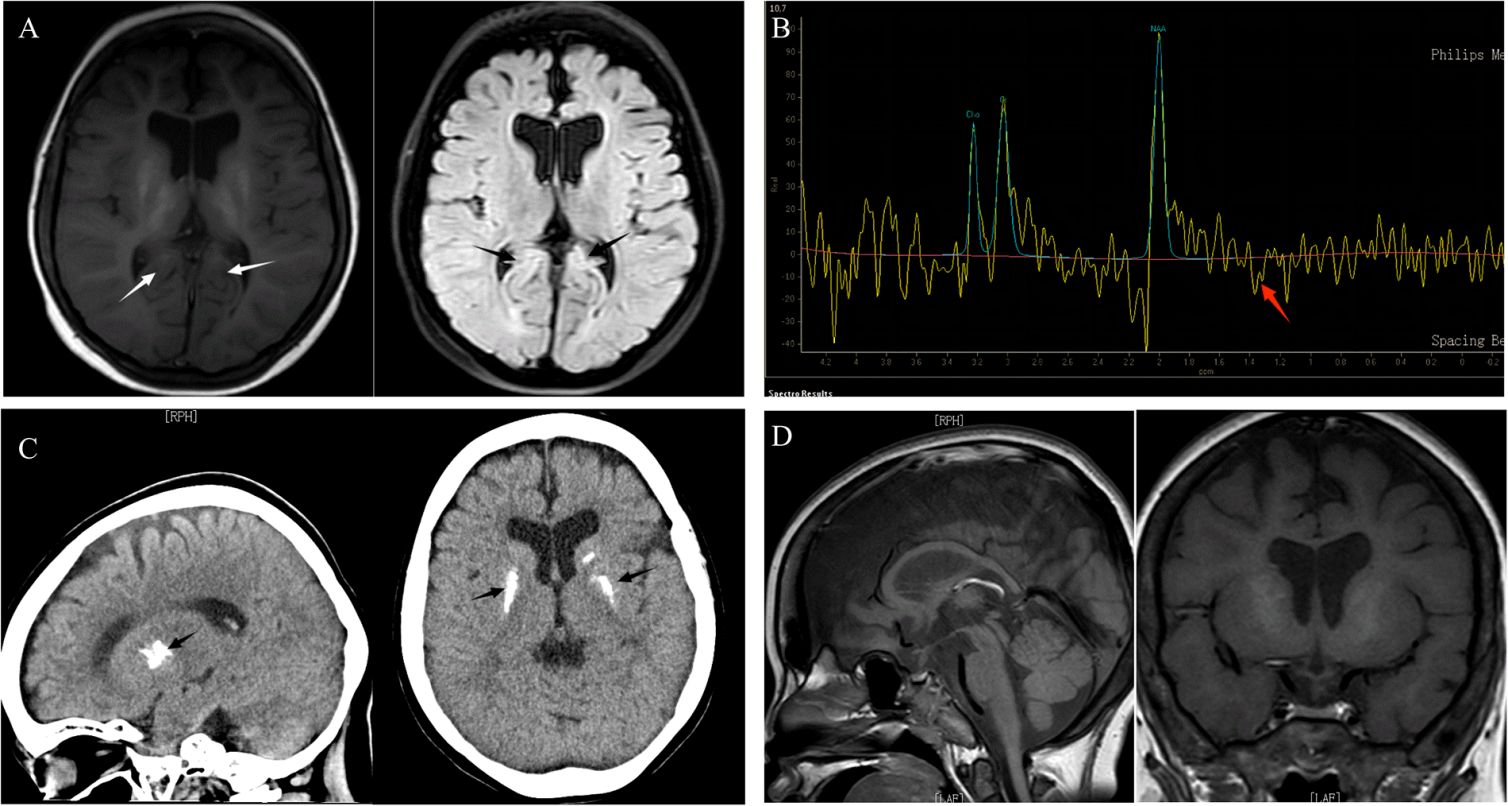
The patient's MRI and brain CT scan findings of brain and pituitary abnormalities. (A) Brain MRI: axial T1, axial T2 FLAIR: On T2-FLAIR, a strip of marginally elevated signal may be observed near to the posterior horn of both ventricles (arrows), indicating enlargement of the two ventricles and shrinkage of the brain's white matter. (B) MRS: mildly increased lactate peak. (C) CT scan: basal ganglia calcifications. (D) Pituitary MRI: the pituitary gland was slightly tiny, measuring 2.60 mm in height, without constriction, with uniform pituitary signal, evident strong signal in the posterior pituitary lobe, and visible pituitary stalk.
